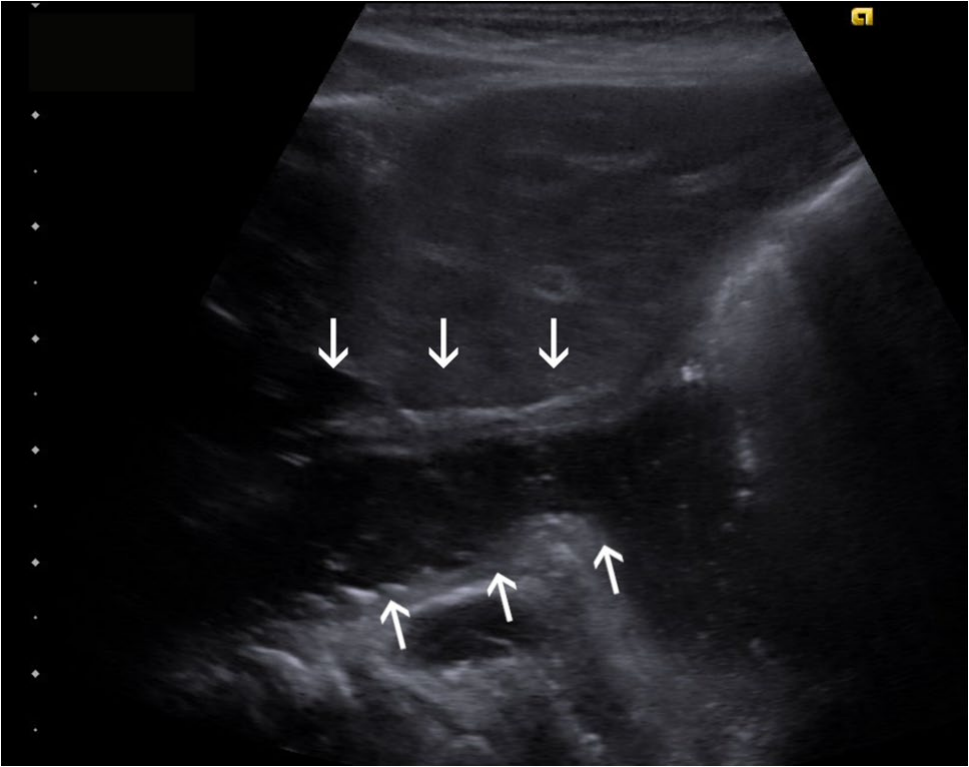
A left oblique parasagittal ultrasound image of the gastroesophageal junction in a 3-month-old boy. The cardia and distal esophagus are wide open (*arrows*) and there is reflux into the distal esophagus
